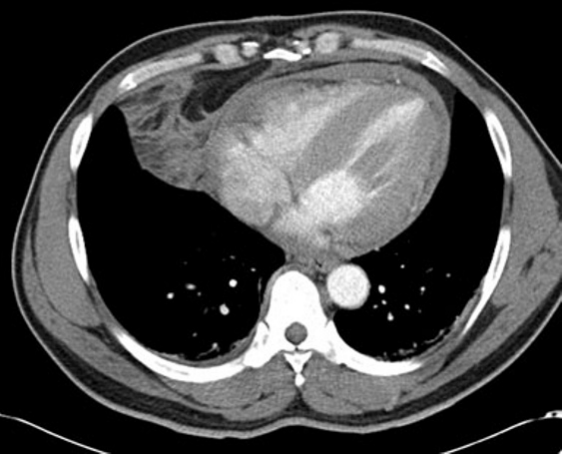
A 49-year-old male who sustained a stab wound to the epigastrium. The CT identified the presence of a pericardial fluid collection (approximate Hounsfield attenuation value of 40) and fat stranding within the epicardial fat. Intra-operatively this patient was confirmed to have a pericardial laceration and haemopericardium
